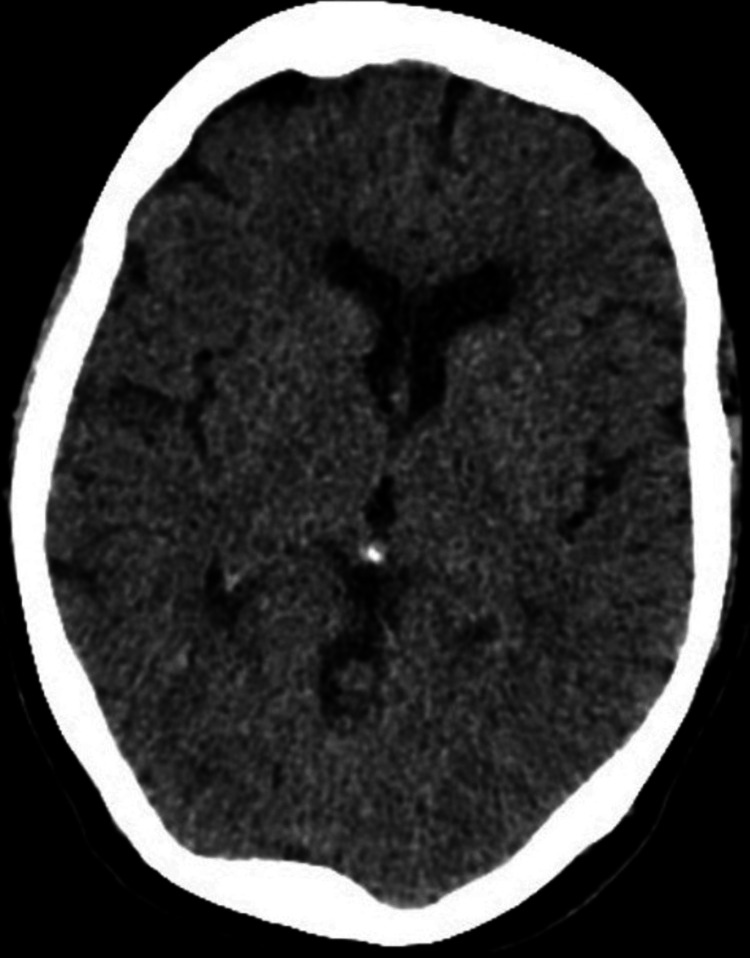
Axial non-contrast CT head showing complete resolution of cerebral venous sinus thrombosis (CVST).
